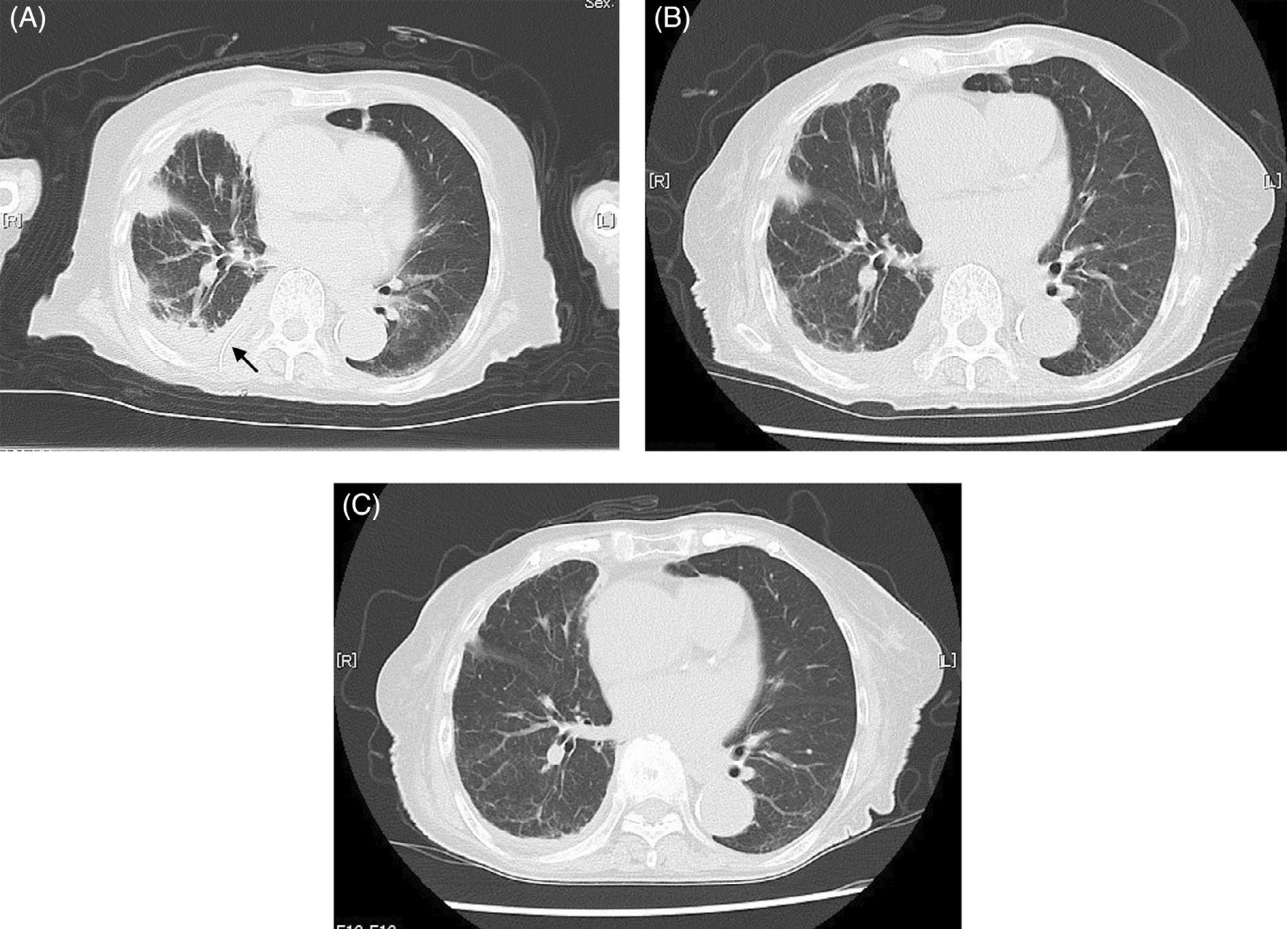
Chest CT scan images captured during pigtail catheter insertion and after drainage. The pigtail catheter was inserted into the cavity of the right lower lobe. (A: pigtail catheter is indicated by black arrow) Subsequent CT images taken 1 month (B) and 2 months (C) after treatment reveal a marked reduction in the pyothorax cavity, leaving only a trace.
